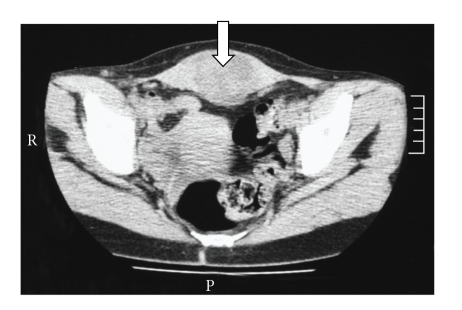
Computed tomograms of the abdomen show a heterogeneous and ill-defined tumor
